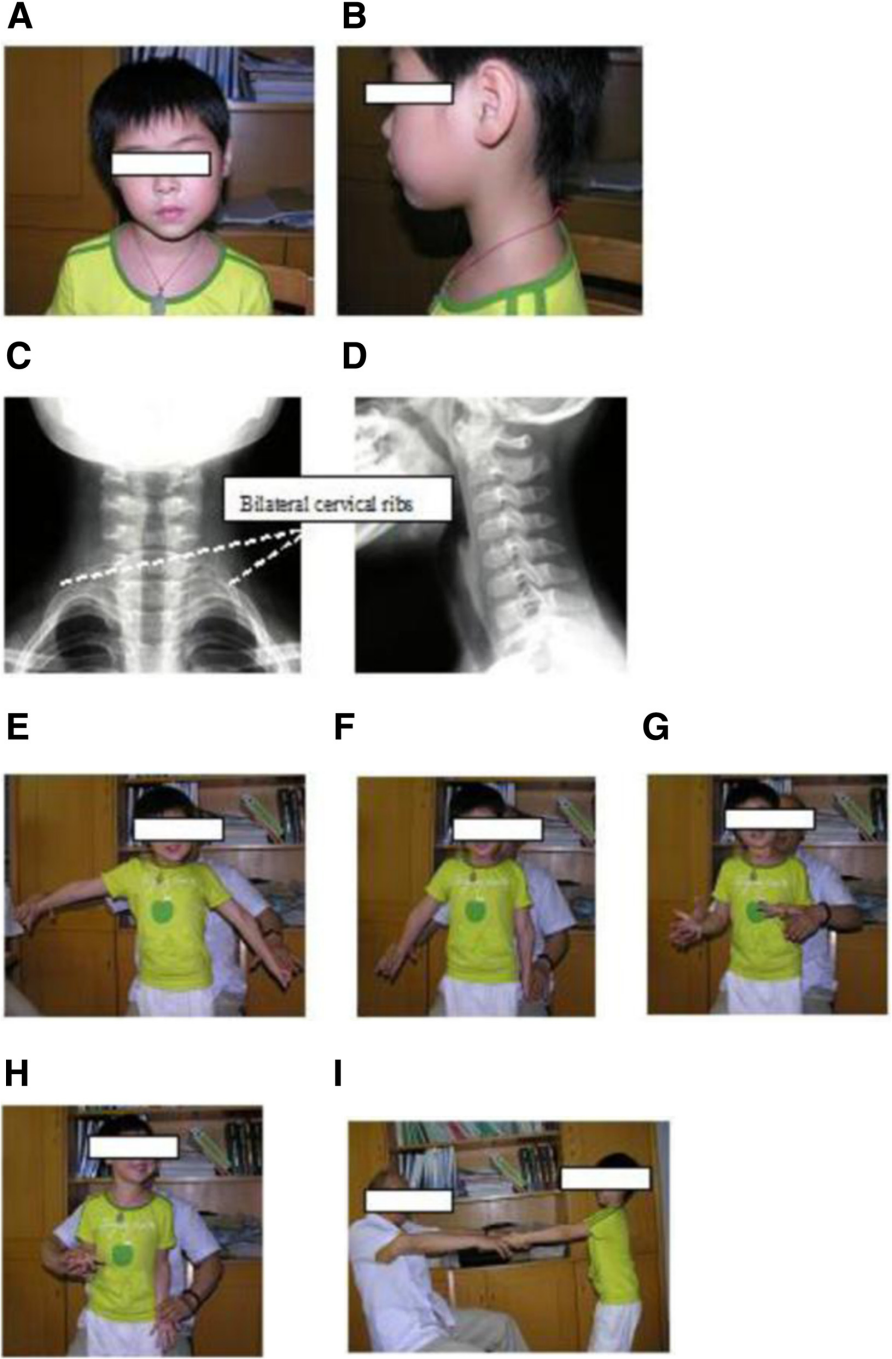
A 6-year-old girl had neck, shoulder and bilateral upper limb pain and discomfort for more than 2 years. She had complained of left upper limb pain since she was 3 years old. (A) On examination, the left side of the girl’s face is obviously larger than the right side and (B) she reported that her neck was stiff. Bilateral cervical ribs can be seen on an anteroposterior X-ray film of the cervical spine (C), and a lateral X-ray film of the cervical spine shows disappearance of the physiological curvature of the cervical spine (D). After receiving a local nerve block in the neck, the girl was alert, agile, and cooperative during physical examination. The shoulder abduction and external rotation strength, elbow flexion strength and hand-clenching strength of the left upper limb were poorer than those of the right upper limb. Wright’s test and Adson’s test were positive, and Hoffmann’s sign was negative. Her left shoulder abduction strength decreased at 90 degrees (E). Her left shoulder abduction strength was decreased at 30 degrees (F), and the left shoulder external rotation strength was also decreased (G). Her elbow flexion strength was decreased (H), as was her hand grip strength (I). After she received a local nerve block, her shoulder, elbow and hand strength increased significantly and the acmesthesia noticeably improved.
